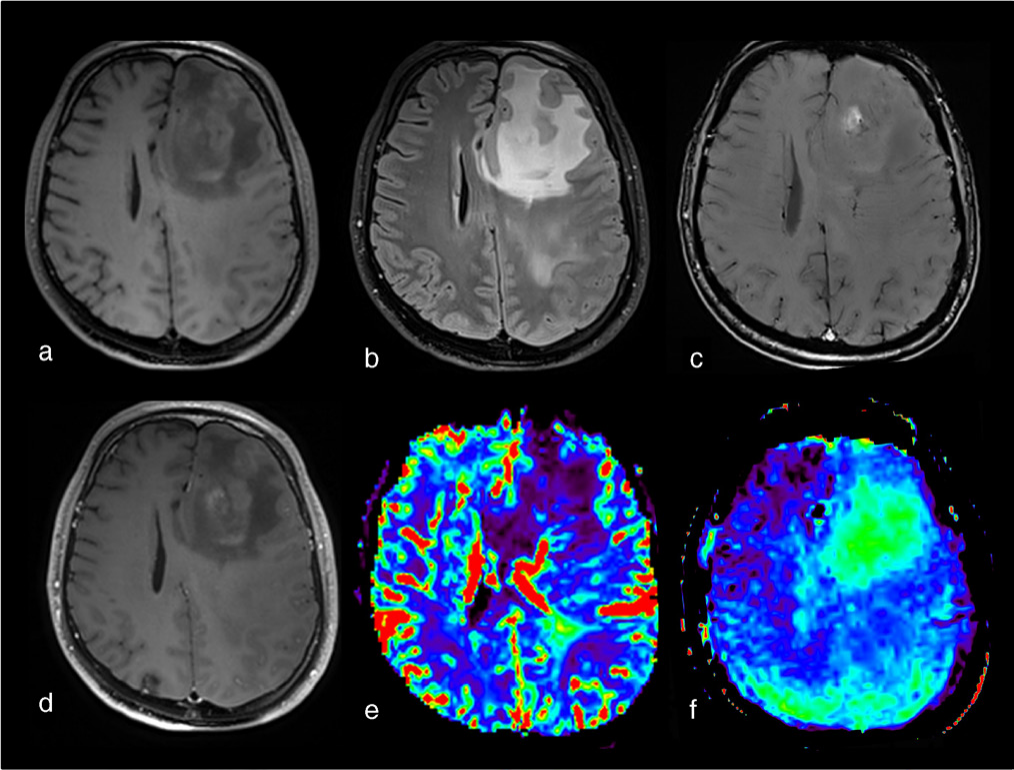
Another example of a non-treated glioblastoma (DH-wildtype, WHO grade 4) that displays high malignant features on the APTw sequence in contrast to DSC perfusion. (a) 3D T1w SE in the axial plane showing an intra-axial lesion with mass effect on brain parenchyma and lateral ventricles. (b) 3D FLAIR. (c) SWI sequence showing some hemorrhagic components. (d) 3D T1w SE injected sequence showing mild homogenous contrast enhancement. (e) rCBV map after leakage correction. (f) A F.S. APTw map. For this case, a 55% duty cycle protocol was used. In the posterior part of APTw image B0 artifact are seen. 3D, three-dimensional; APTw, amide proton transfer-weighted; cNAWM, contralateral normal-appearing white matter; FLAIR, fluid attenuated inversion recovery; ROI, region of interest.
